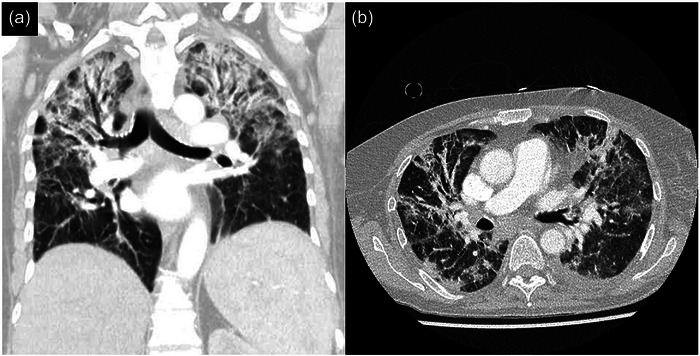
Chest computed tomography with (a) coronal and (b) axial views showing bilateral upper lobe infiltrates on day 38 of hospitalization.
